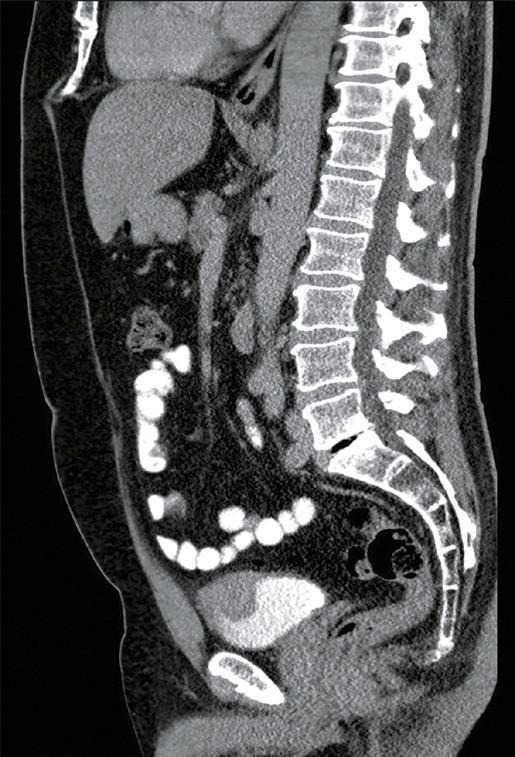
Computed tomography scan in the lateral projection before surgery (tumor indicated by the arrow)
